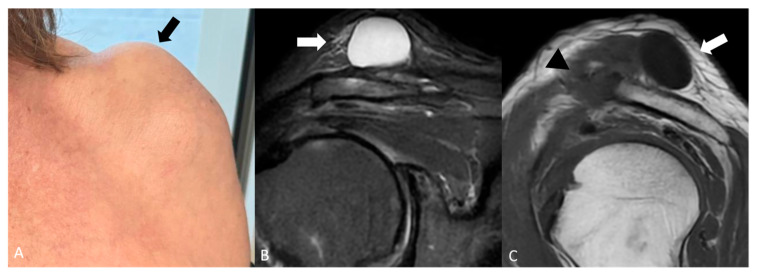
(A) Elderly woman with a soft bump on the cranial portion of her right shoulder (black arrow). (B) coronal STIR and (C) sagittal T1-weighted showing a cystic lesion superior to the acromion-clavicular joint (white arrows). The lesion is raising subcutaneous soft tissue. Acromioclavicular osteoarthritis is noted (black arrowhead).
